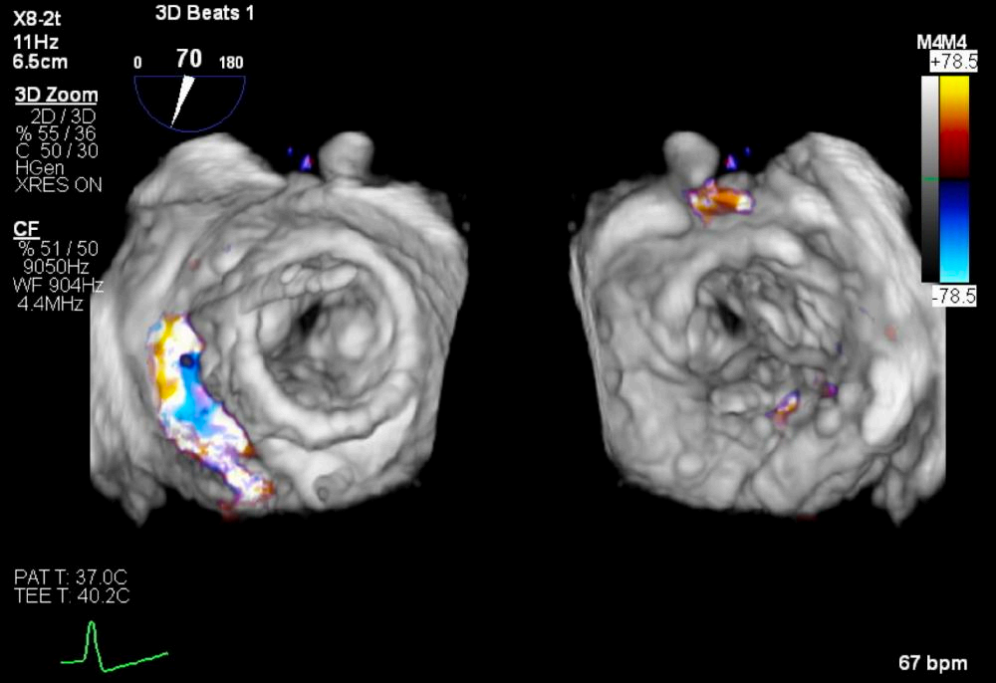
Residual paravalvular leak in 3D echocardiogram.
